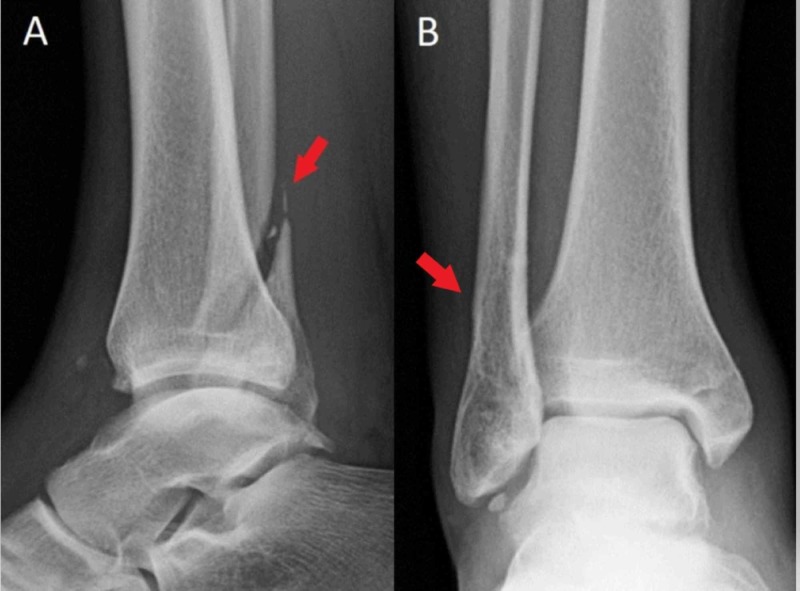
Successful closed reduction Lateral (A) and anteroposterior (B) radiographs demonstrate successful closed reduction after the described technique. Reduction of the tibiotalar joint with widening of the fibular fracture is shown (red arrows). Medial malleolar fracture remains minimally displaced. The patient was splinted after closed reduction.
